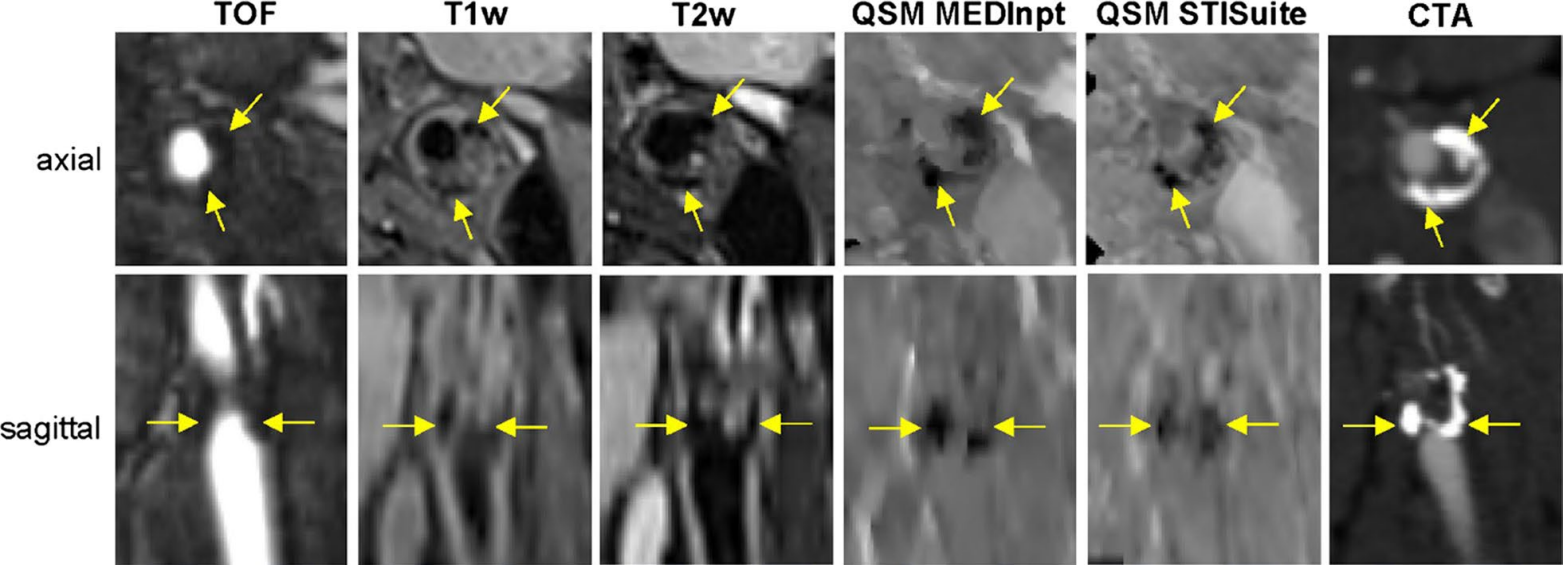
Quantitative susceptibility mapping (QSM) of a carotid plaque. Example of magnetic resonance imaging of a heavily calcified plaque almost fully occluding the left internal carotid artery at the level of carotid bifurcation with hypointense appearance on time-of-flight (TOF) and black-blood T_1_weighted (T_1_w) and T_2_-weighted (T_2_w) images as well as strongly negative susceptibility on QSM images computed with MEDInpt and the STISuite. The computed tomography angiography (CTA) image acquired 1.5 years before CMR at 0.6-mm resolution from the same patient is shown to help with plaque localization (Reprinted with permission from: Nguyen et al. [82])
